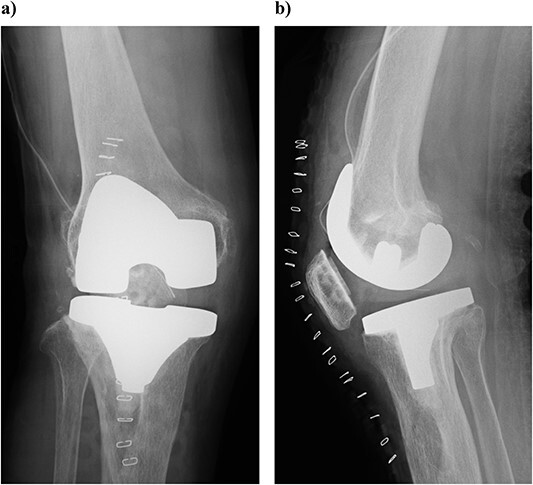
Plain radiography immediately after the surgery: (a) front and (b) lateral side. A slight dilation of the medial joint space is observed.
